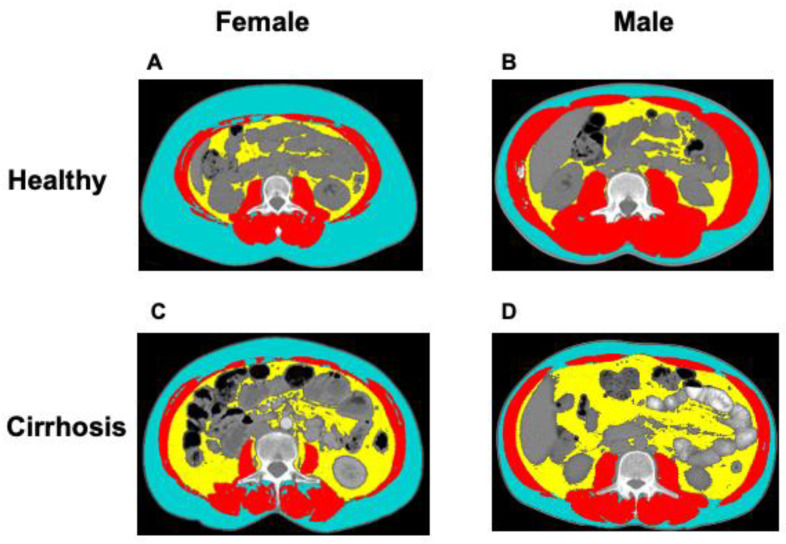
Abdominal computed tomography (CT) images taken at the 3rd. lumbar vertebra of healthy donors and patients with cirrhosis applied for body composition assessment. Example of body composition features in a (A) female healthy donor, (B) male healthy donor (C) female patient with cirrhosis, and (D) male patient with cirrhosis. Muscle, subcutaneous, and visceral adipose tissue are shown in red, blue, and yellow, respectively.
